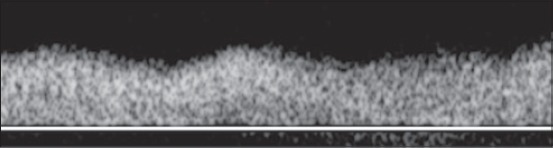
Pulsed-wave Doppler image of monophasic waveform of a portal vein as an example.
